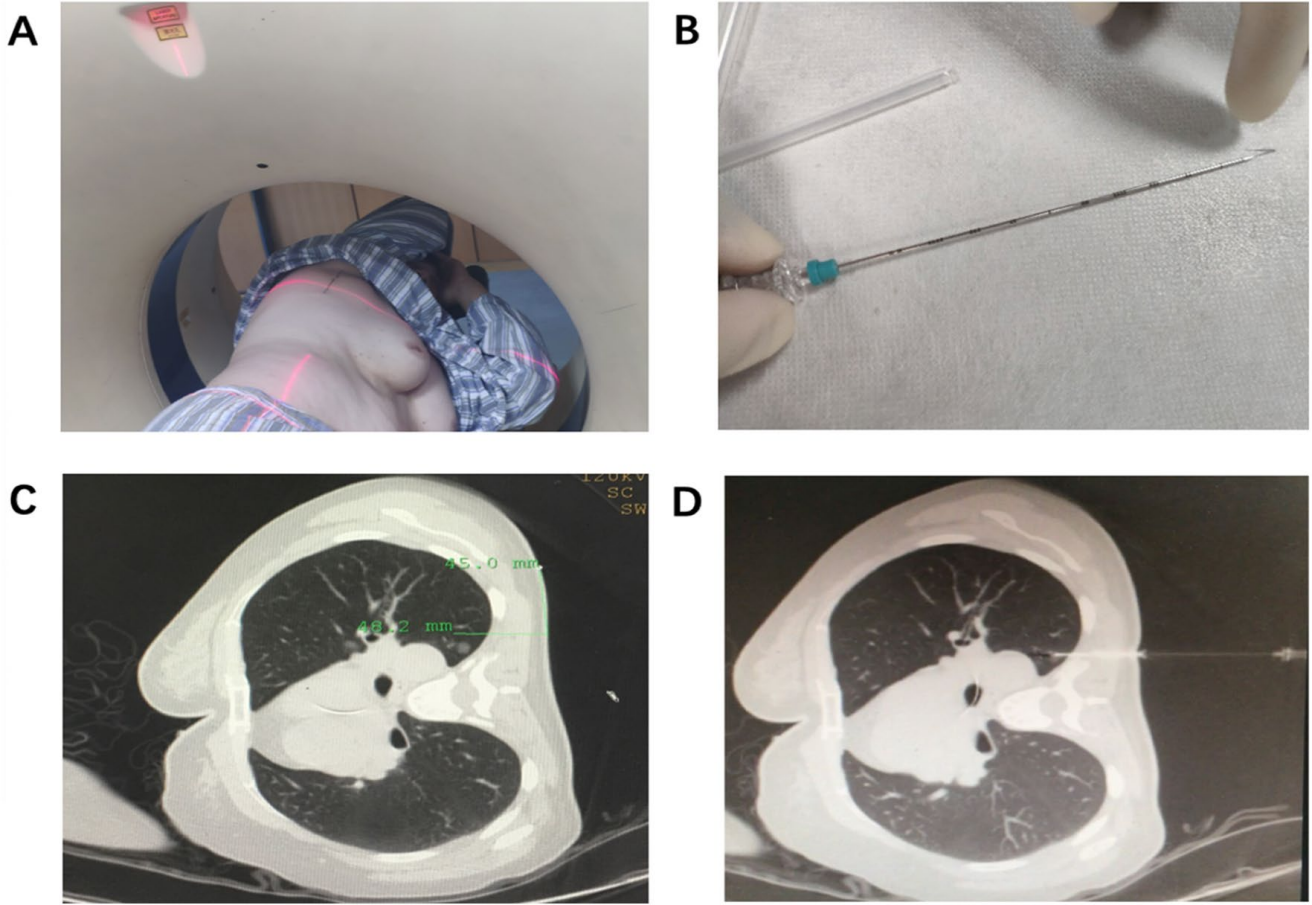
(A) Coronal localization by CT and Sagittal marking with steel needles. (B) The picture of steel needle. (C) Measuring the distance of puncture point and locating the distance between steel needle and puncture point in coronal position of CT image. (D) The results of puncture were confirmed by CT and the complications were detected.
